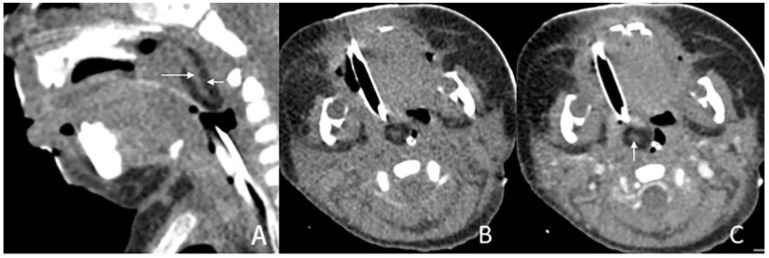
CT of a 1-month and 16-day girl presenting with throat congestion 29 days after birth. (A,B) Sagittal and axial CT, displayed in the soft-tissue window, shows a sausage-like fat density mass (short arrow) with a soft tissue density core (long arrow) originating from the left lateral pharyngeal wall. (C) Axial, postcontrast CT, displayed in the soft-tissue window, shows that the lesion’s stalk is slightly enhanced (arrow), but the rest of the lesion is not enhanced.
